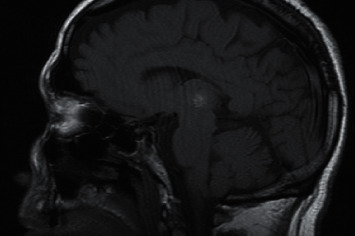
Magnetic resonance imaging where we can observe hemorrhagic transformation in the left thalamus of the infarction.
